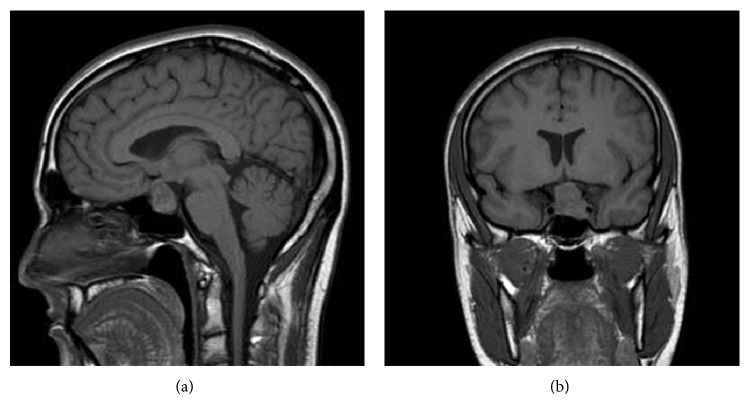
MRI, without contrast, revealed an enlarged sellar lesion on (a) sagittal T1-weighted and (b) coronal T1-weighted images. The optic chiasm cannot be clearly visualized in the coronal T1-weighted image.
